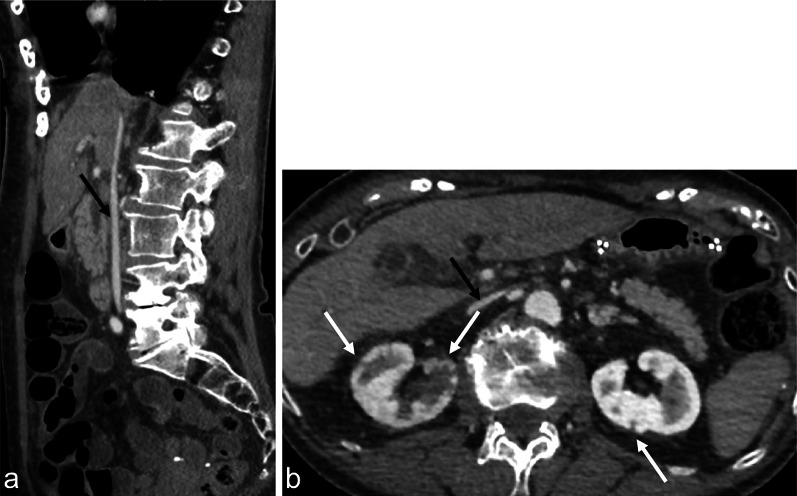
Contrast-enhanced CT in the portal venous phase, showing an 84-year-old male with sepsis (qSOFA 2) due to bilateral nephritis (white arrows). A collapsed IVC can be observed in both the sagittal (a, black arrow) and axial views (b, black arrow)
